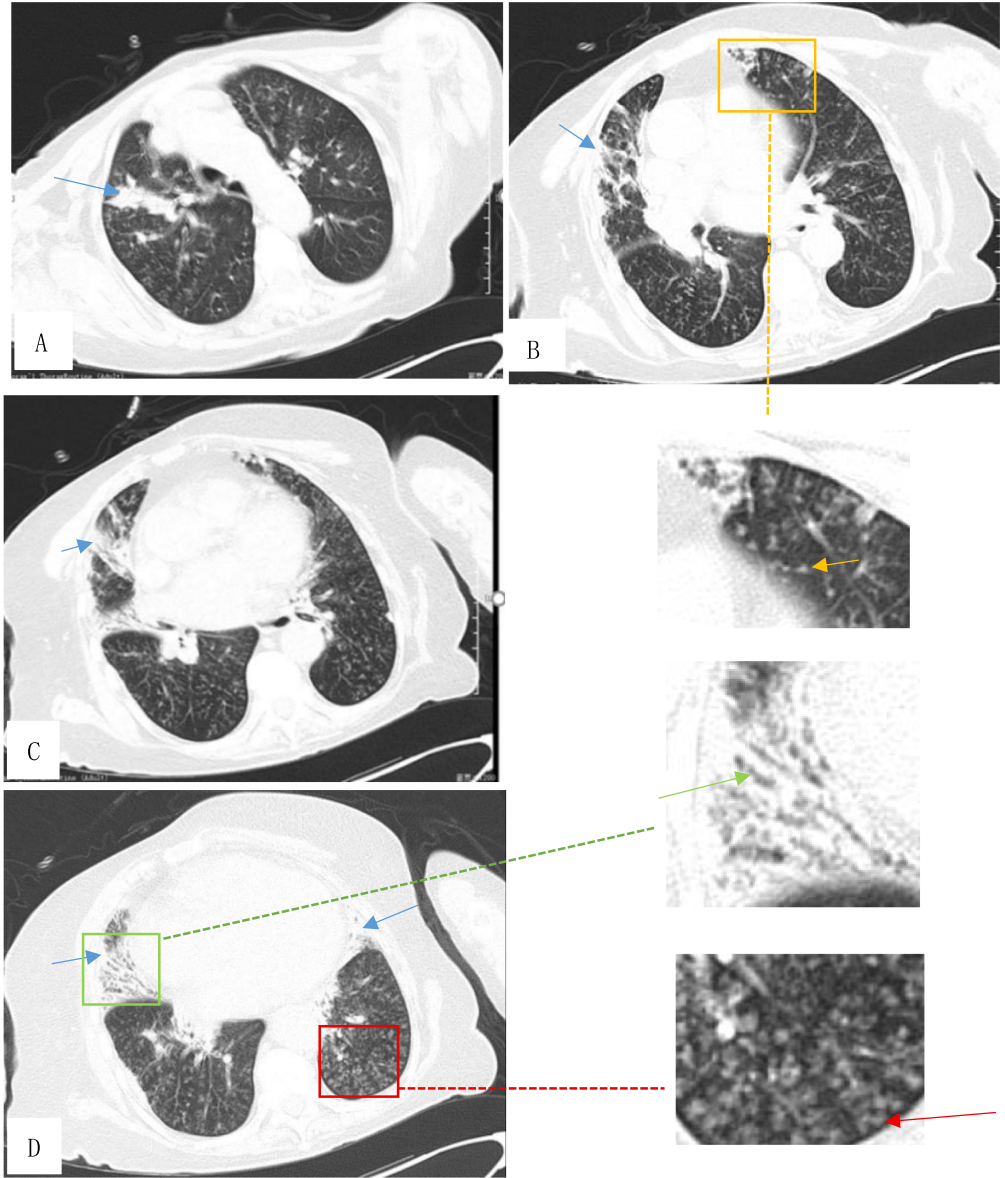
(A–D) D1 HRCT of the chest showed diffuse lung lesions: diffuse miliary nodules opacities (orange arrow) and “tree‐in‐bud” patterns (red arrow) distributed throughout both lung fields, infiltration shadows (blue arrow) in both upper lungs and the middle lobe of the right lung, as well as traction bronchiectasis (green arrow) in the right middle lobe.
